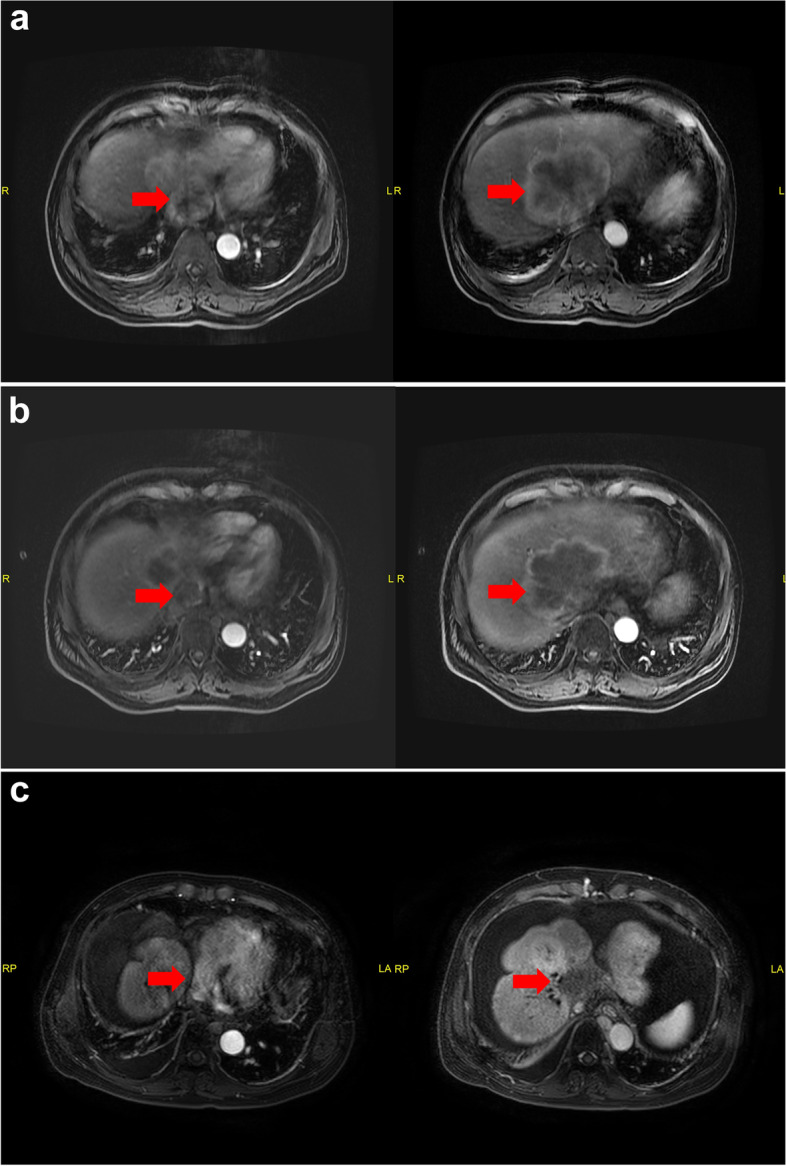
The classic patient (Case 9) with IVCTT and RATT received SBRT. January 2018: The primary upper-abdominal MRI scan with lesions a MRI scan in May 2018 b and March 2022 c after SBRT. The patient died of infectious shock due to hepatic failure in May 2022
